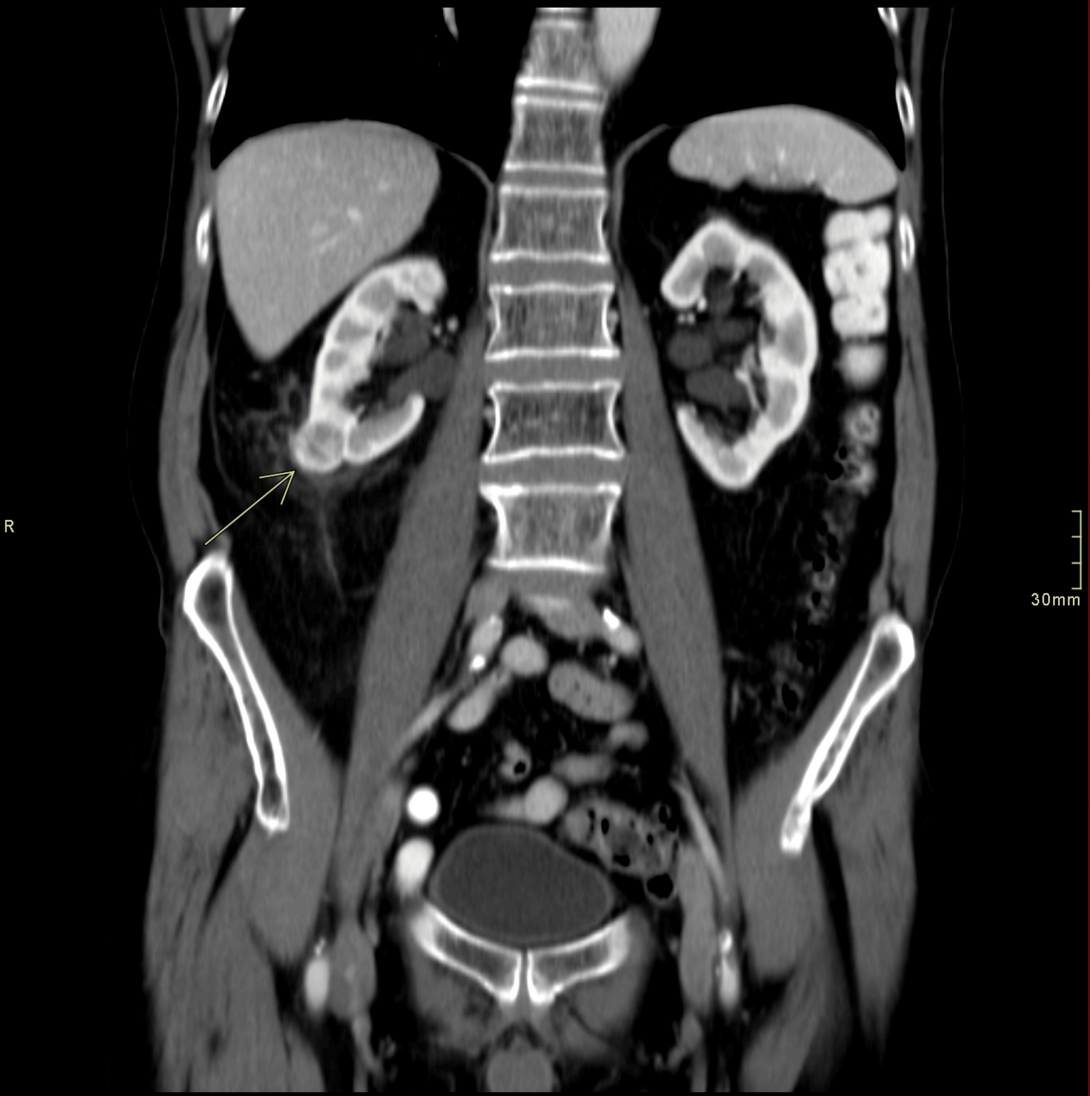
Coronal abdomen and pelvis CT scan with IV contrast/abdomen window showing a 2.0 x 2.3 cm exophytic right lower pole heterogeneously enhancing lesion consistent with an oncocytoma The arrow points to the kidney lesion
